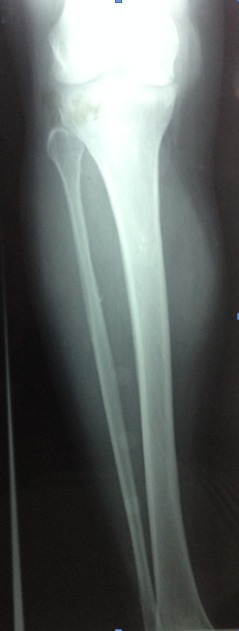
X-ray of the right leg: increased density of soft tissue in the medial aspect of the tibia
